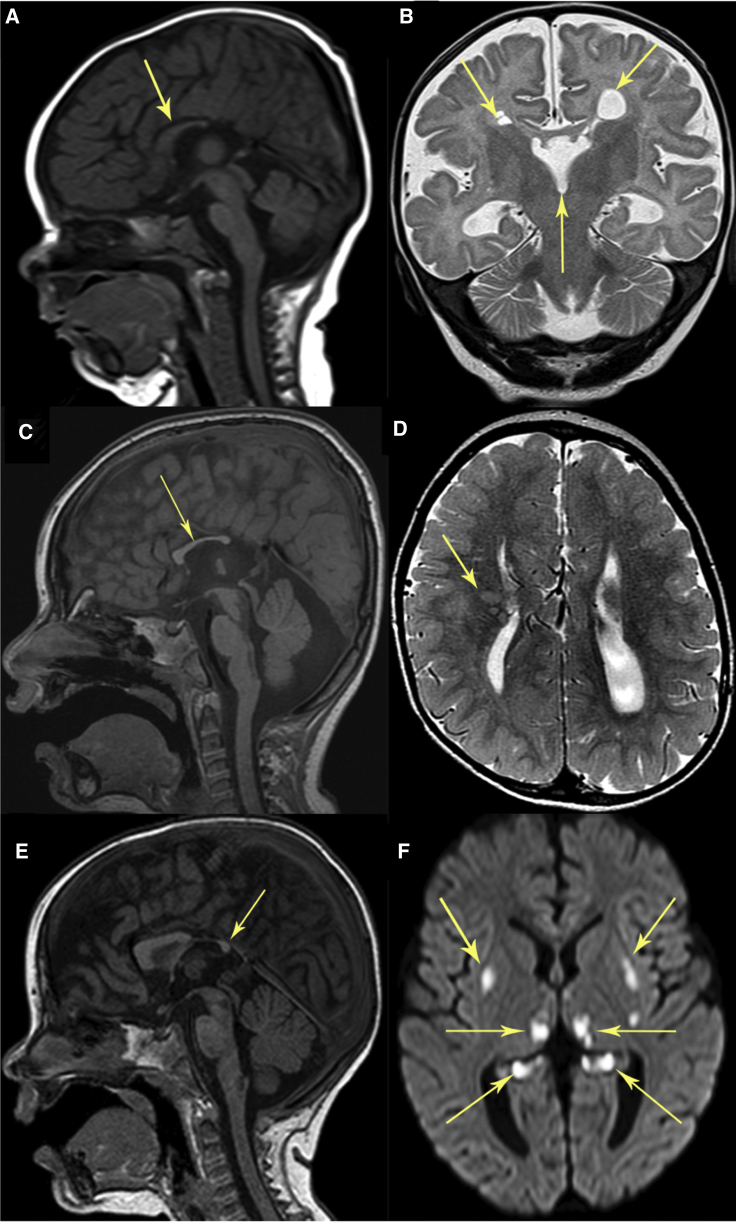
Neuroimaging of Subjects Harbouring Bi-Allelic Pathogenic *NDUFAF8* Variants (A and B) Subject 1 at 5 months of age. (C and D) Subject 2 at 2 years, 9 months of age. (E and F) Subject 3 at 1 year, 2 months of age. Sagittal T1-weighted imaging (T1WI) (A, C, and E) demonstrates dysmorphic corpus callosum (arrows): For subjects 1 and 2, no splenium is present (A and C), and only a small posterior part of the splenium is present in subject 3 (E). Sagittal T1WI (C and E) reveals signal abnormality in the dorsal brainstem corresponding to areas with restricted diffusion for subjects 2 and 3. Coronal T2-weighted imaging (B) of subject 1 demonstrates bilateral periventricular cysts and absent septum pellucidum (arrows). Axial T2-weighted imaging (D) depicts right frontal gray matter heterotopia in subject 2 (arrow). Axial diffusion-weighted imaging (F) shows bilateral symmetrical diffusion restriction in the putamina, thalami, and hippocampal tails in subject 3 (arrows).
